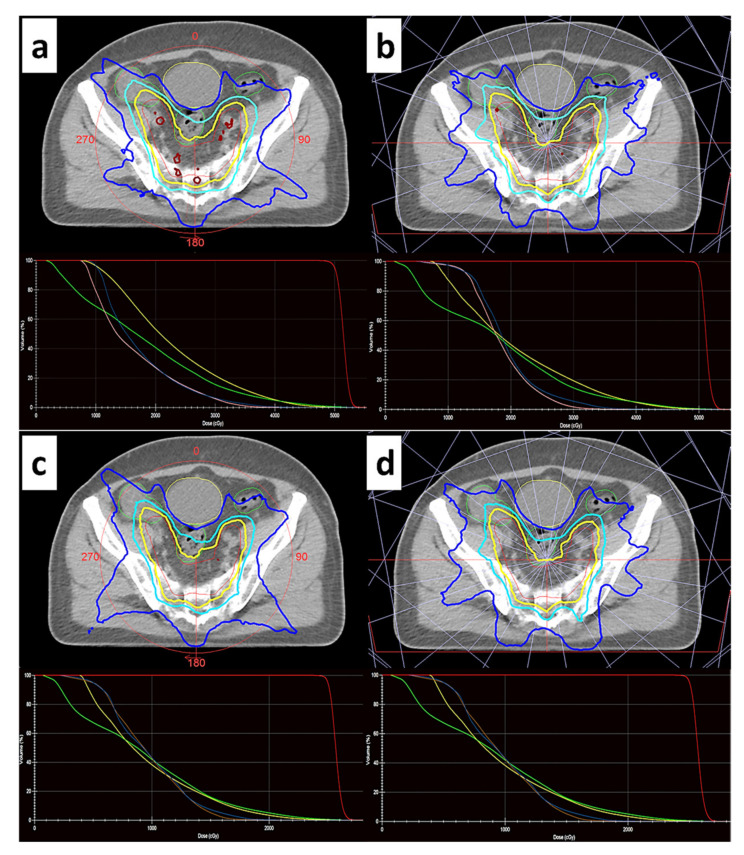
For an indicative patient, an axial slice of the planning CT scan is shown with isodose lines superimposed, for the (a,b) CFRT (25 × 2 Gy) and (c,d) HFRT (5 × 5 Gy) fractionation schemes. Corresponding cumulative DVHs are given below. (a,c) VMAT treatment delivery technique. (b,d) IMRT treatment delivery technique. The photon beam energy of 10 MV is used for all plans shown. Isoline legend: dark blue: 50%; light blue: 75%; yellow: 95%. DVH and contour legend: red: PTV; yellow: bladder; green: bowel; pink: left femoral head; blue: right femoral head.
